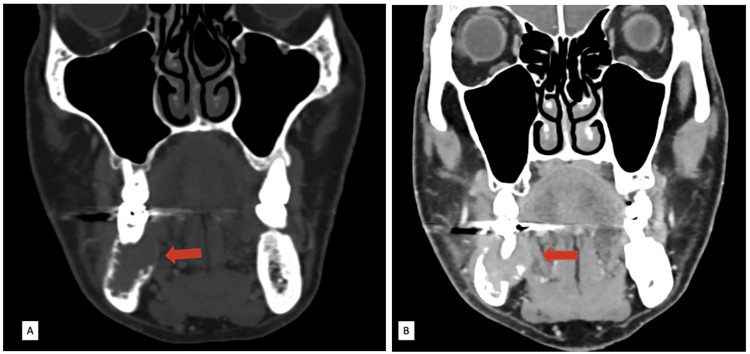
Contrast-enhanced CT neck images in the coronal plane. (A) Bone window setting showing the expanded right hemimandible with cortical erosion (red arrow). (B) Soft tissue window setting showing the soft tissue mass extending to the lingual surface of the gingiva (red arrow).
